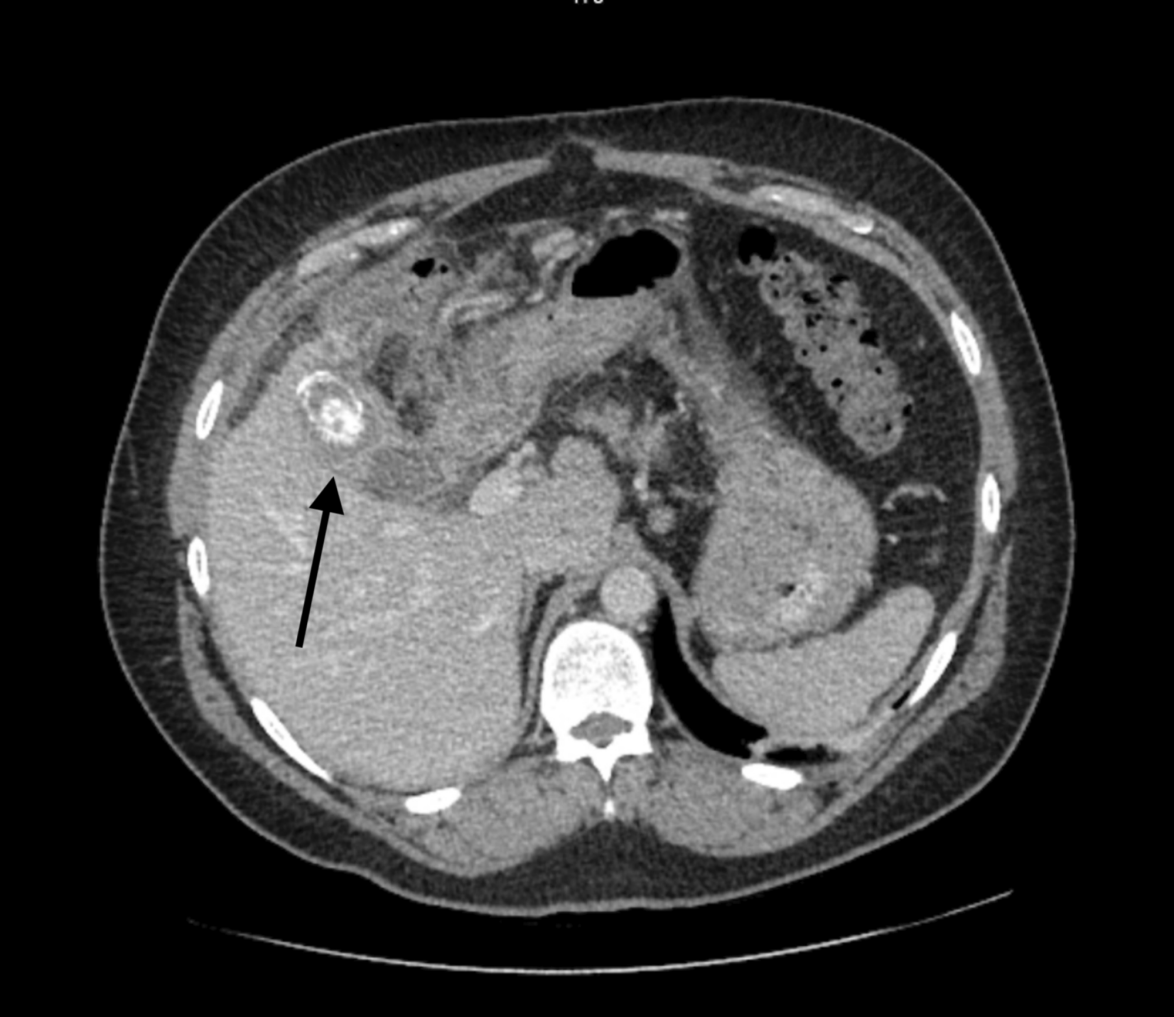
CT scan with significant inflammatory changes in the right upper quadrant, surrounding a gallstone; malignancy cannot be ruled out.
